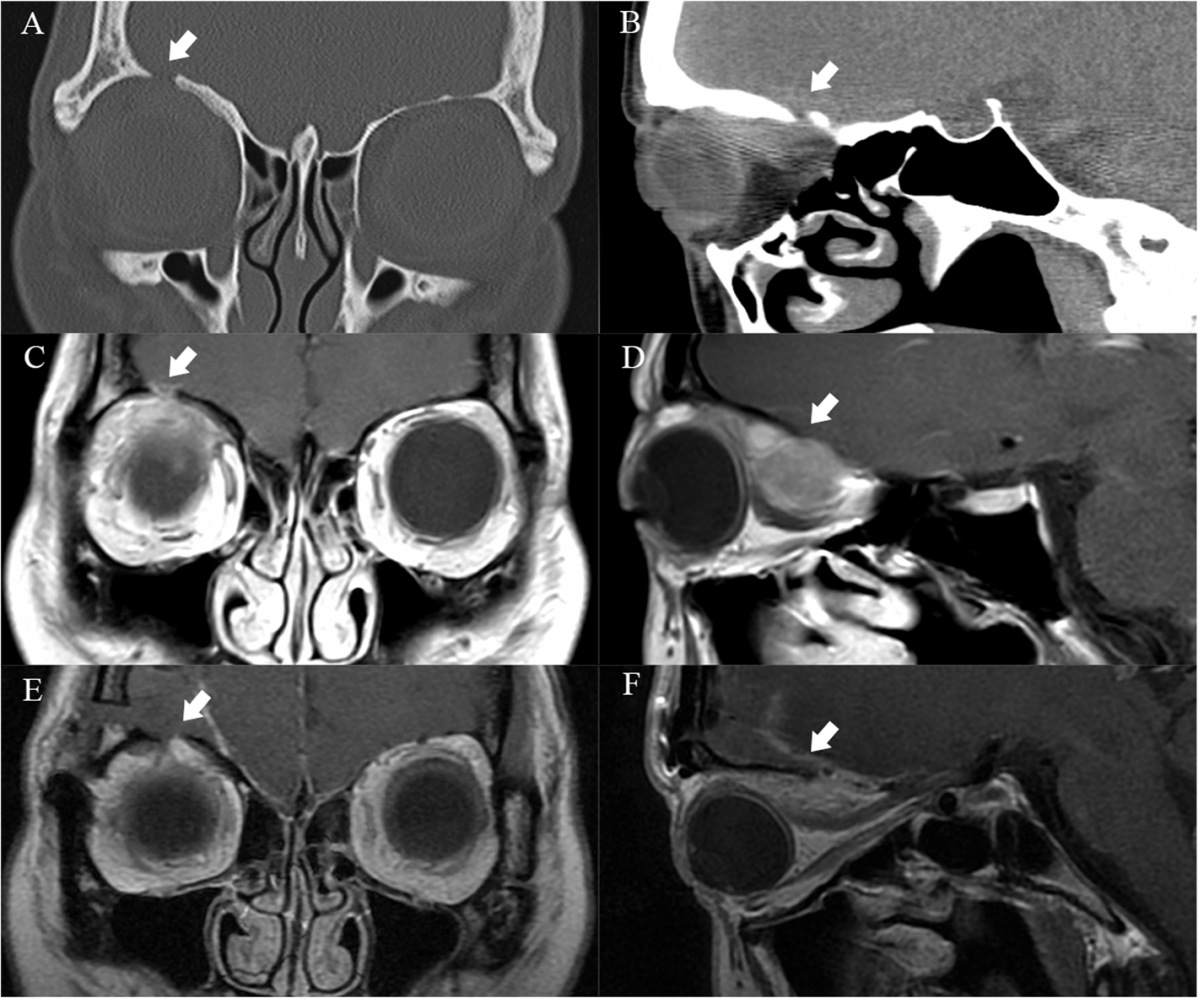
Case 5. The patient was a 46-year-old with a chronic disease process and right exophthalmos for more than 3 months. The patients underwent 2 operations (Transorbital approach) 12 years ago and 6 years ago. a, b: CT showed that the tumor involved the bone of supraorbital bone with bone destruction (arrow). C, D: MRI showed that the main body of the tumor invaded the intracranial dura through the destruction of the skull base bone (arrow). e, f: After the Transfronto-orbital approach was given to the craniotomy, the MRI re-examinations immediately after the operation showed that the tumors were removed completely. There was no recurrence after 5 years of MRI follow-up
